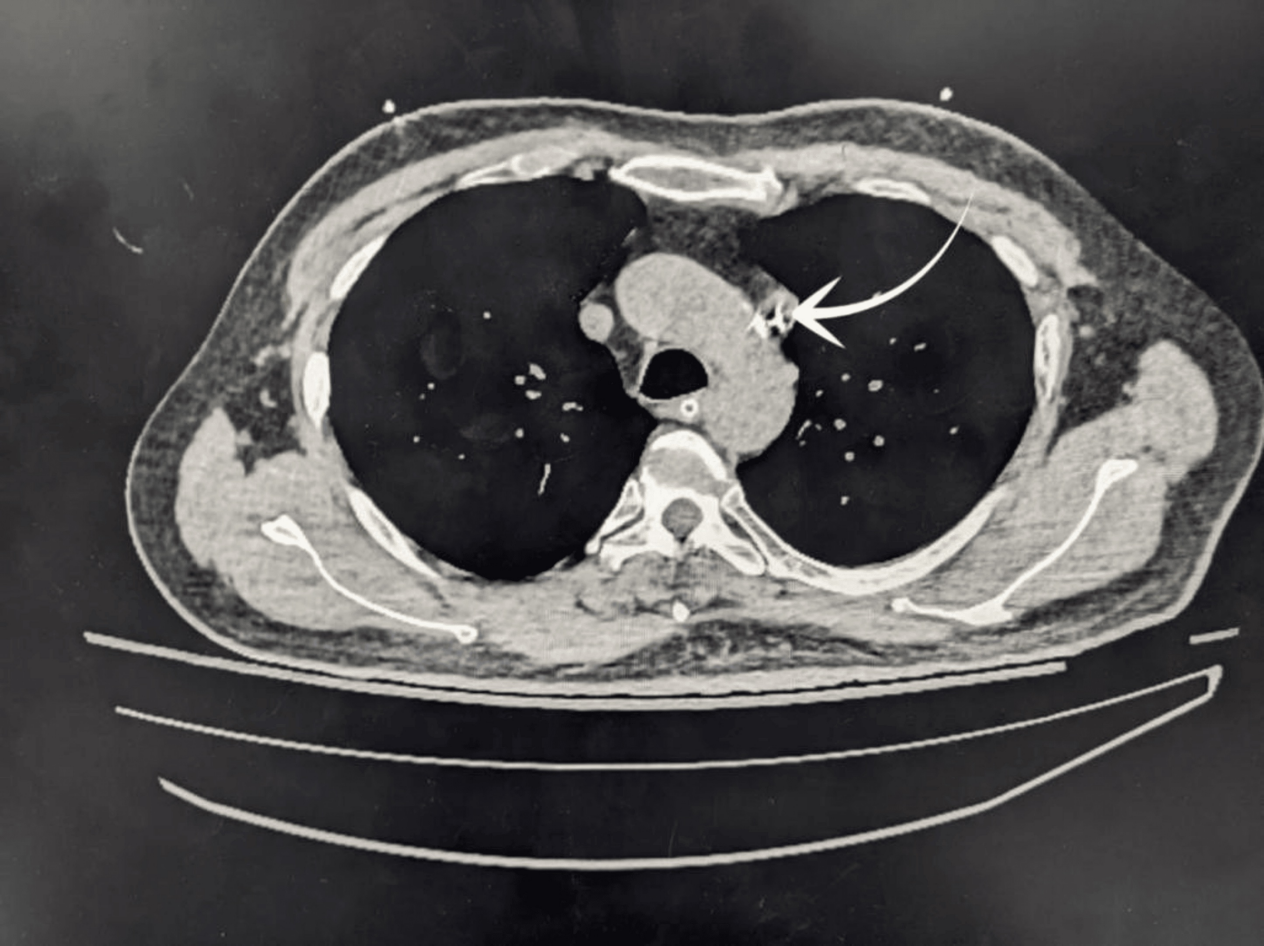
CT chest (axial view) showing bilateral superior vena cava (SVC) with central venous pressure (CVP) in the left SVC pointed by an arrow
